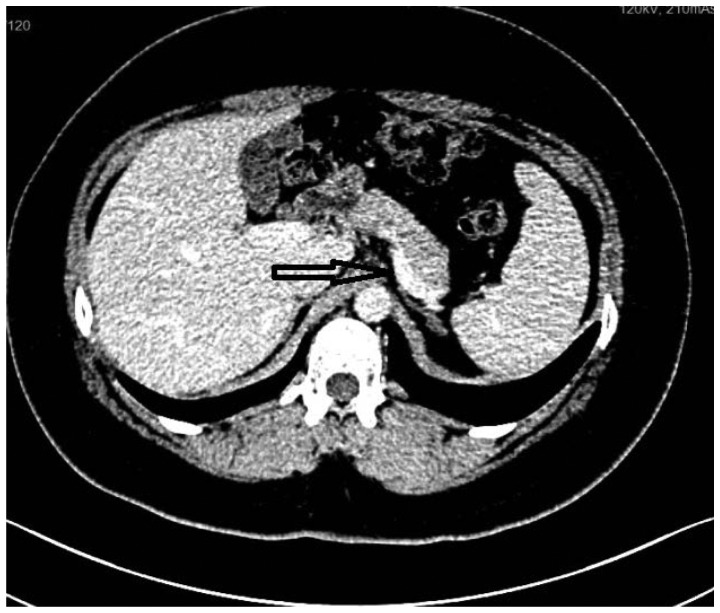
Computed tomography scan of the pancreas. An enhanced lesion was observed around the tail of the pancreas, which was well-defined, homogeneously distributed and had dimensions of ~2.1×1.1 cm, indicating a possible benign lesion.
